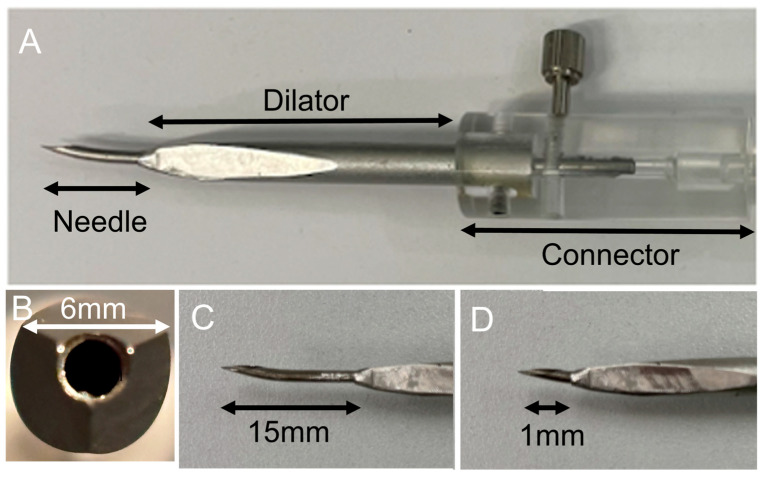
Ultrasound-guided cricothyroidotomy kit. (A): Image showing the developed ultrasound-guided cricothyroidotomy kit. The kit includes a 16-G Tuohy needle, dilator, and connector. (B): Dilator features a 6 mm diameter structure with a triangular pyramid tip and three cutting edges. The connector links the needle to the dilator and the screw connector adjusts and secures the length of the needle exiting the dilator. (C): Effective puncture length of the needle was fixed at 15 mm. (D): When the screw connector was completely loosened, the needle was shortened to 1 mm from the tip. However, the needle did not enter the dilator even when the screw connector was entirely loosened.
